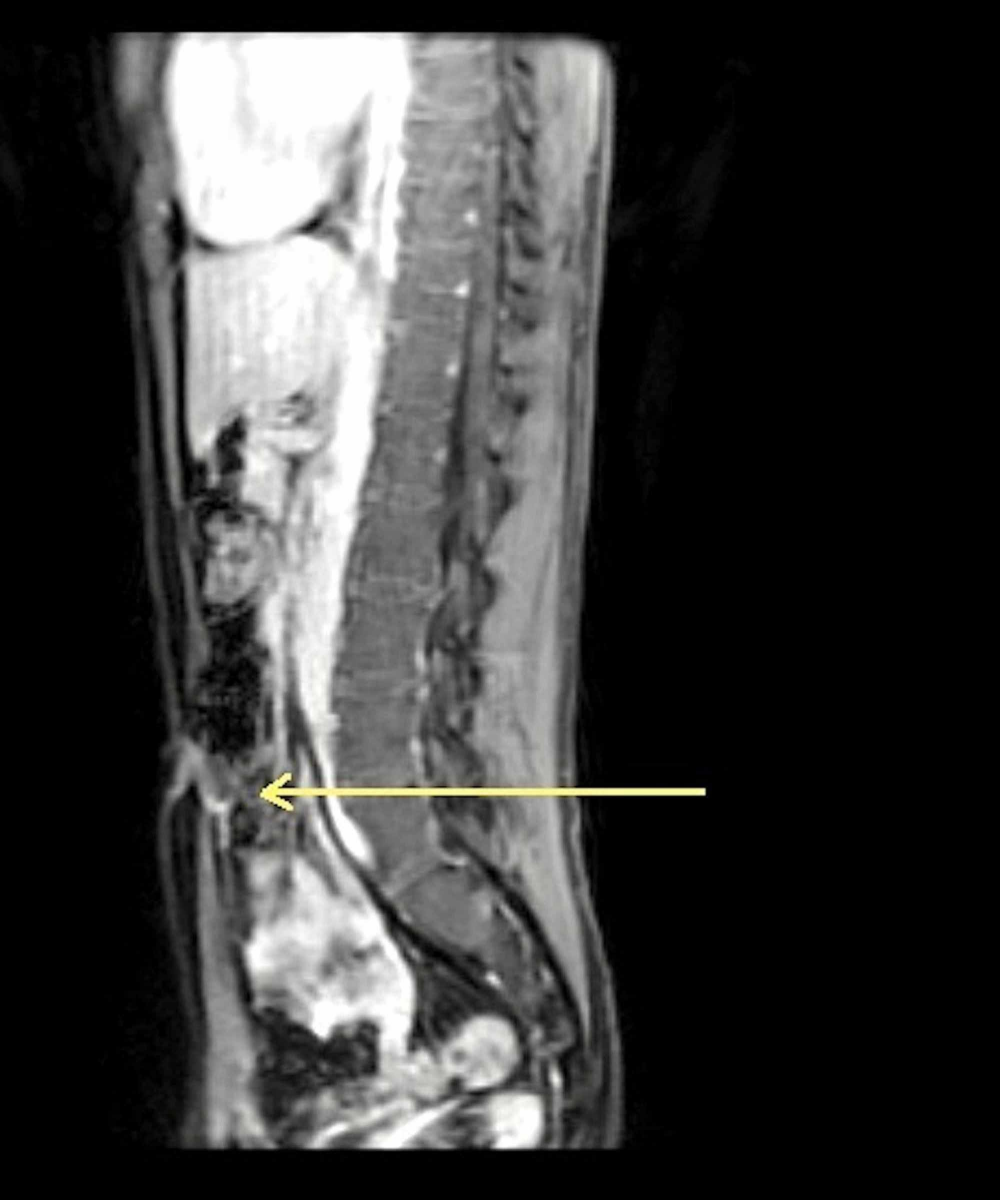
An MRI scan of the abdomen and pelvis delineating a urachal sinus (arrow) complicated by abscess formation.
